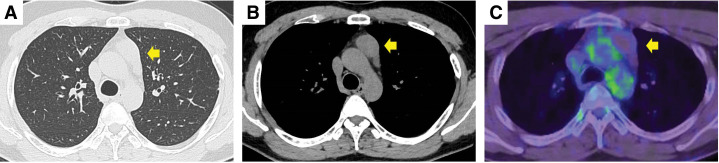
Preoperative image. Chest computed tomography shows a 35 × 25 mm mass on the left side of the anterior mediastinum in the lung (A: arrow) and mediastinal windows (B: arrow). Positron emission tomography with 18-F-fluorodeoxyglucose demonstrated no accumulation in the mass (C: arrow).
